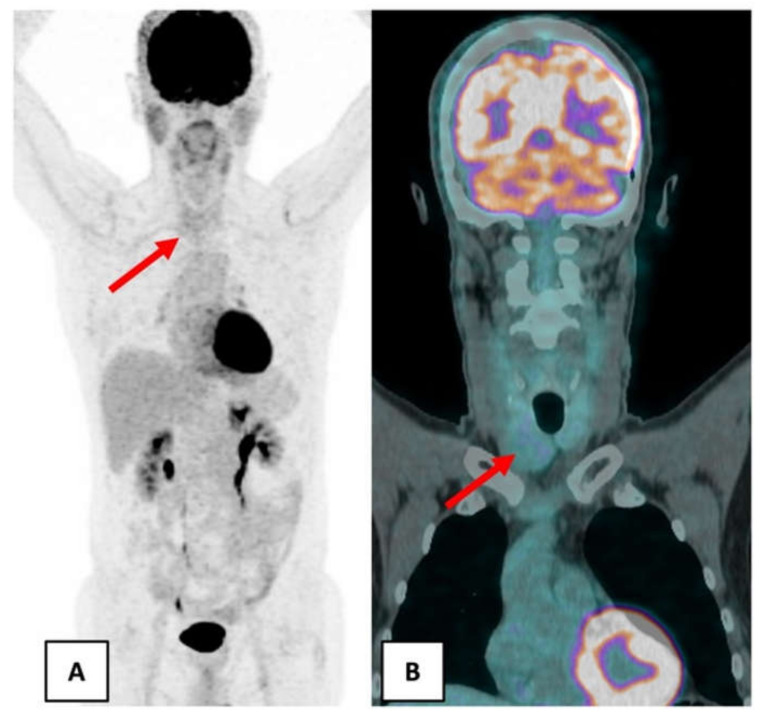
June 2018 ^18^F-FDG PET/CT showed a right lobar thyroid nodule without significant hypermetabolism (red arrow). (A) Maximum intensity projection. (B) Coronal fusion PET/CT.
